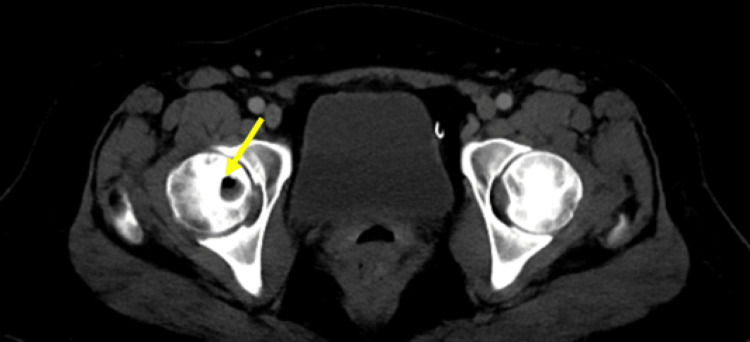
Femoral head osteonecrosis on CT. Earliest detection of avascular necrosis of the femoral head in abdomen/pelvis CT scan. Note the large necrosis in the head of the right femur (arrowhead).
